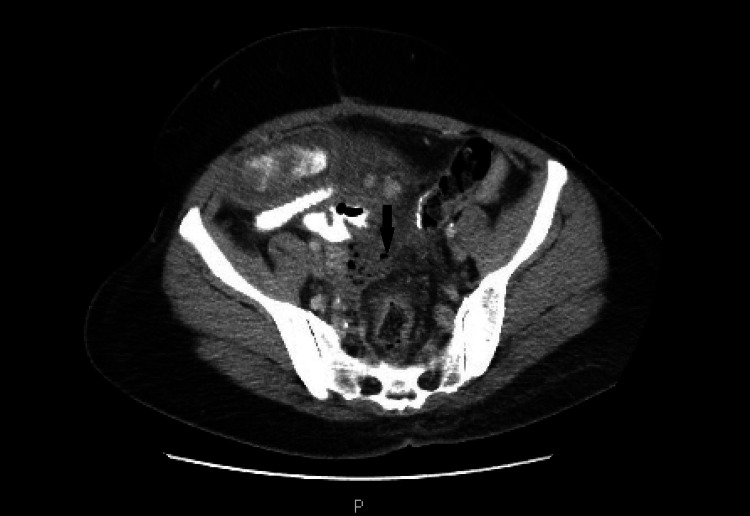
Computed tomography of the abdomen showing dilatation and wall thickening of a long segment of distal small bowel in the right anterior hemiabdomen with multiple surrounding foci of intraperitoneal air (arrow)
